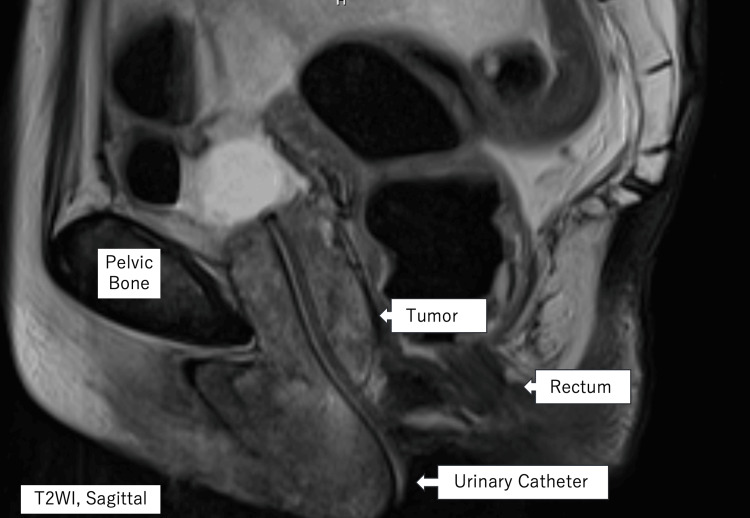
Pelvic MRI at initial presentation A T2-weighted sagittal MRI of the pelvis shows an oval-shaped tumor occupying the entire length of the urethra.
